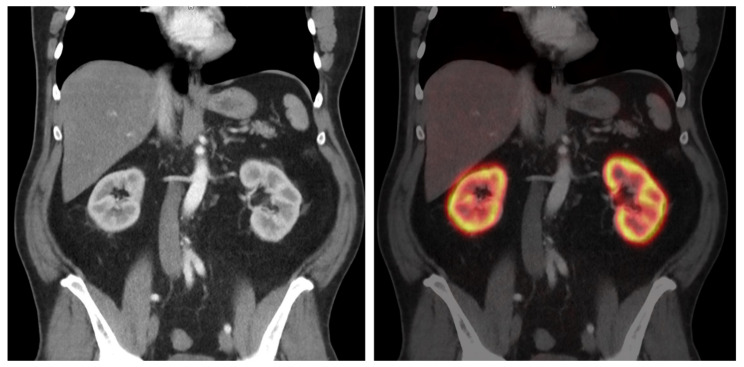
Example of renal PSMA ligand uptake in [^68^Ga]Ga-PSMA-11 PET/CT. (Left): Contrast-enhanced CT scan with visual differentiation between renal cortex and medulla in arterial phase. (Right): [^68^Ga]Ga-PSMA-11 PET/CT fusion with specific uptake of the radiotracer within the renal cortex. Low uptake in other abdominal organs.
